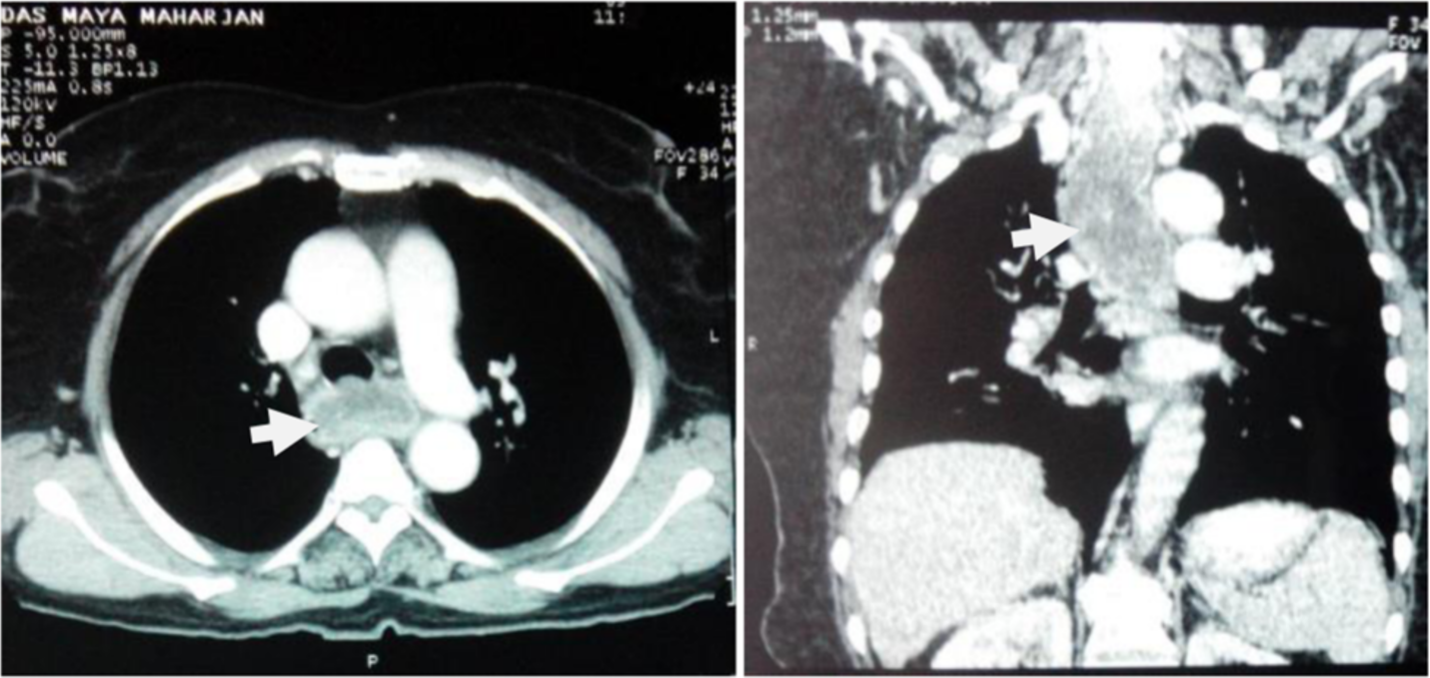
CT images: arrow showing the esophageal mass
